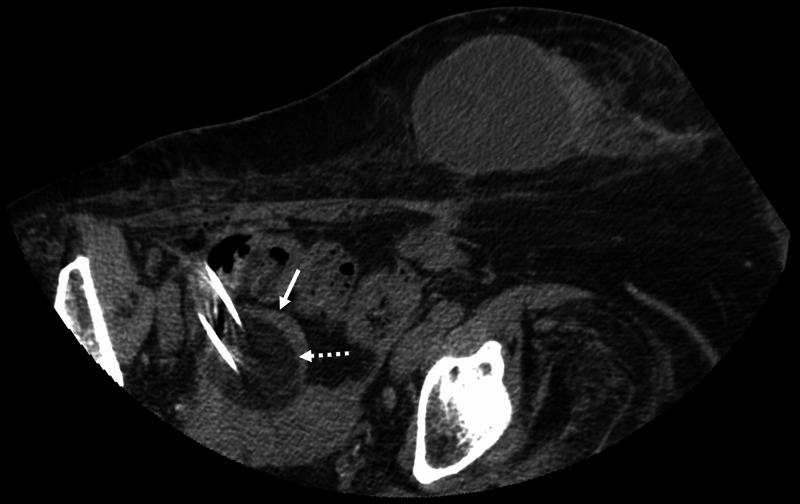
CT scan of the pelvis at the time of the cryoablation procedure Axial non-contrast CT obtained during cryoablation demonstrates positioning of two cryoprobes within the right adnexal mass (solid white arrow) along with formation of a hypodense “ice ball” encompassing the lesion (dashed white arrow).
